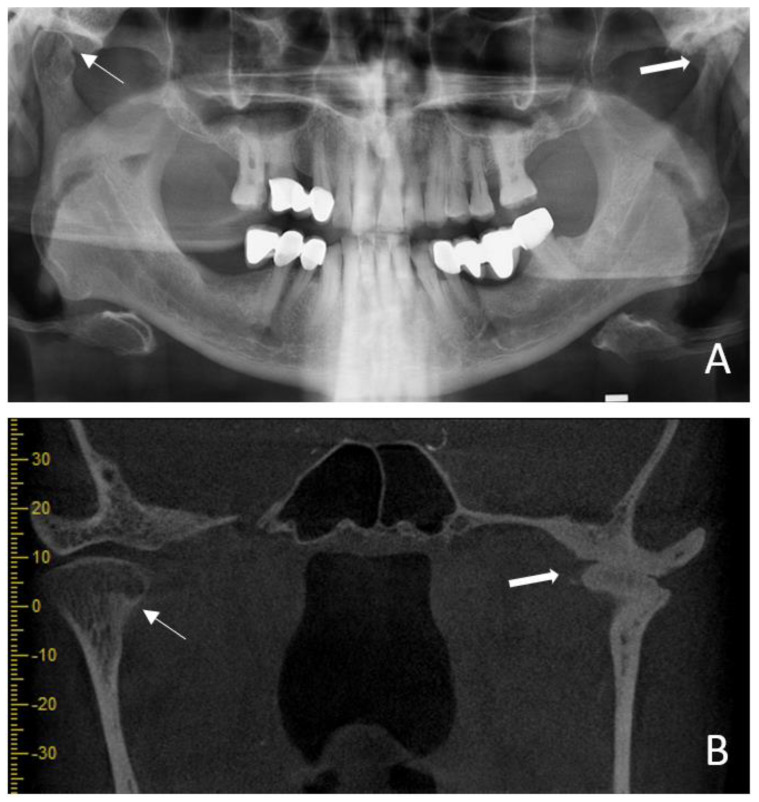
(A) presents the panoramic radiographic image of a 62–year–old patient diagnosed with rheumatoid arthritis for the past 12 years. Resorption at the anterior part of the mandibular condyle (indicated by a thin white arrow), multiple radiopacities at the condylar head, condylar flattening and a decrease in the joint space (indicated by a thick white arrow) are observed. (B) displays the coronal cone beam computed tomography (CBCT) image of the same patient. Resorption at the anterior part of the mandibular condyle (indicated by a thin white arrow), multiple radiopacities at the condylar head and ankylosis (indicated by a thick white arrow) are observed. The findings are consistent with those observed in panoramic radiography, although the actual extent of the disease can be better determined.
